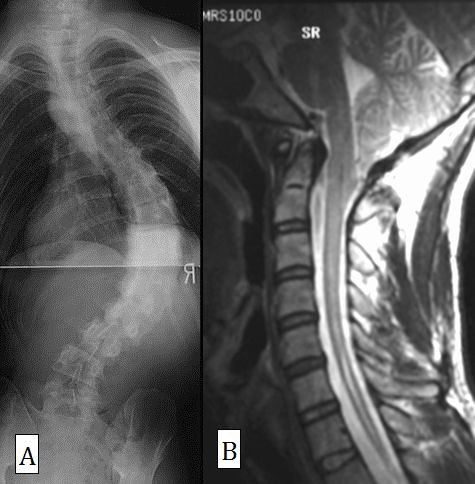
Posteroanterior radiograph (A) and sagittal MRI (B) in Case 1. This patient had bilateral hyporeflexia of SAR and declined neurological decompression surgery, but anterior fusion was performed without any neurological complication. The Cobb angle was 90° and curve flexibility was 52%. The syrinx was slit type.
